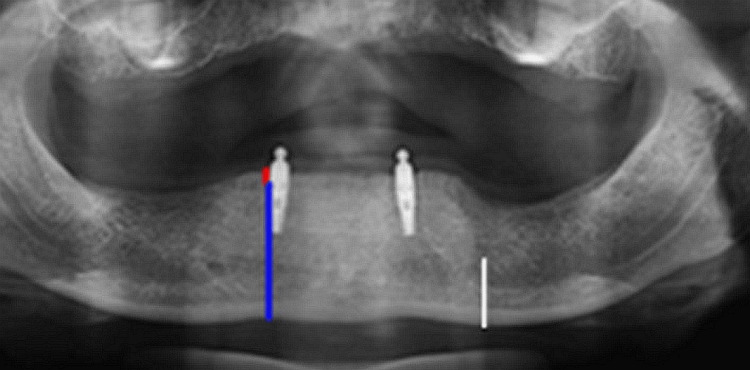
OPG showing the blue, red, and white line. Blue line represents length from crestal bone to the base of mandible (L). Red line represents the rate of resorption (R). White line represents the length from mental foramen to the base of mandible (x). OPG, orthopantomogram
